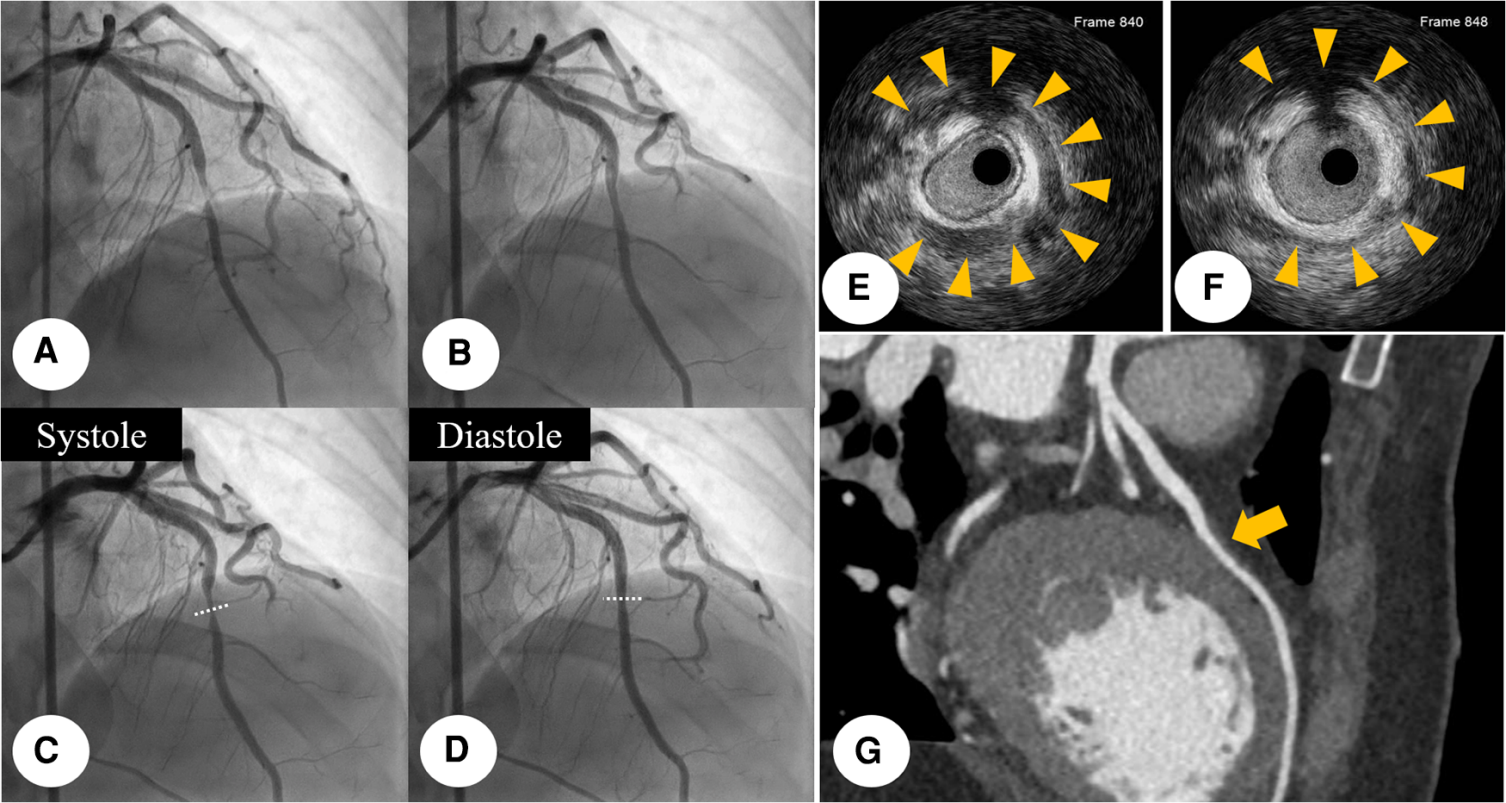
Initial CAG finding: initial CAG demonstrated near-total occlusion (yellowish arrow) at the middle portion of LAD with reduced antegrade coronary flow. (A) This stenosis was dramatically relieved (yellowish arrow), after I-NTG administration (B) After I-NTG administration, systolic compression was seen at the middle portion of LAD, which means MB. (C,D) IVUS was further examined with a guidance system (Eagle Eye® Platinum RX Digital IVUS Catheter, Volcano Corporation, Rancho Cordova, CA, USA), demonstrating eccentric compression with a half-moon-like echo-lucent space between the MB segment and epicardial tissue. (E,F) Multiple-slice CCTA imaging showed a bridged coronary segment surrounded by myocardium at the middle portion of LAD (yellowish arrow). (G) CAG, coronary angiogram; CCTA, coronary computed tomography angiography; I-NTG, intracoronary nitroglycerin; LAD, left anterior descending coronary artery; MB, myocardial bridge.
